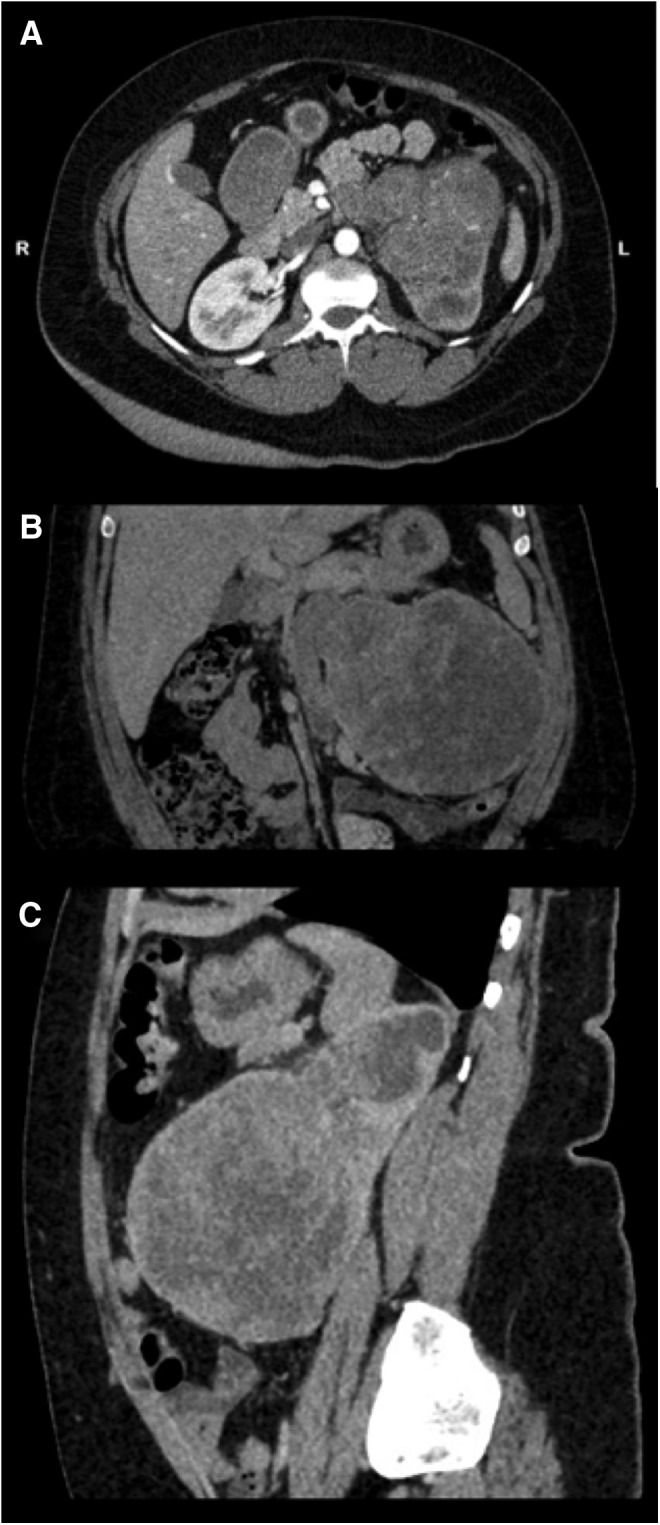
Axial (A), coronal (B), and sagittal (C) view of the contrast-enhanced abdominal CT scan showing a large, heterogeneously enhancing soft tissue mass arising from the lower pole of the left kidney.
